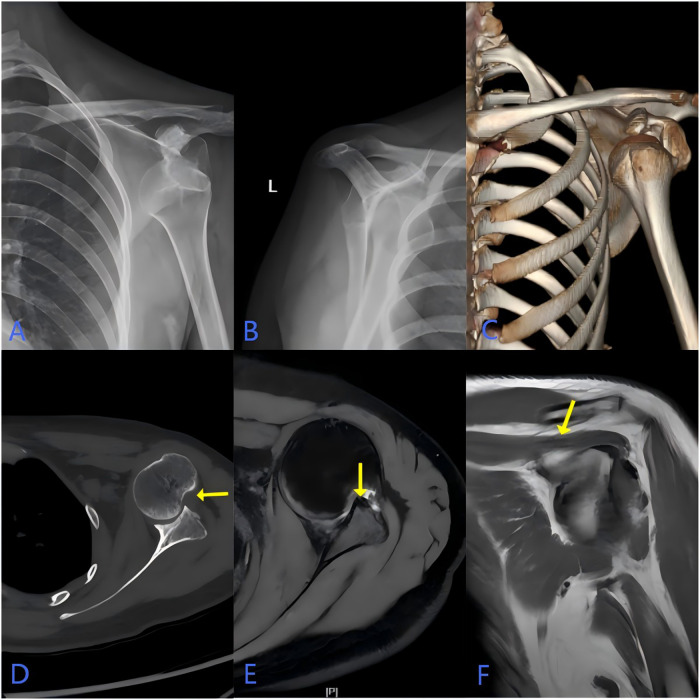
(A,B) preoperative x-ray examination revealed an anterior dislocation of the shoulder joint. (C,D) Preoperative CT scan images revealed a shoulder joint dislocation and a Hill-Sachs lesion in the humeral head (pointed by yellow arrow). (E,F) Preoperative MRI scan revealed a Bankart lesion (pointed by yellow arrow in 2E), and thinning of the rotator cuff tendons (pointed by yellow arrow in 2F).
